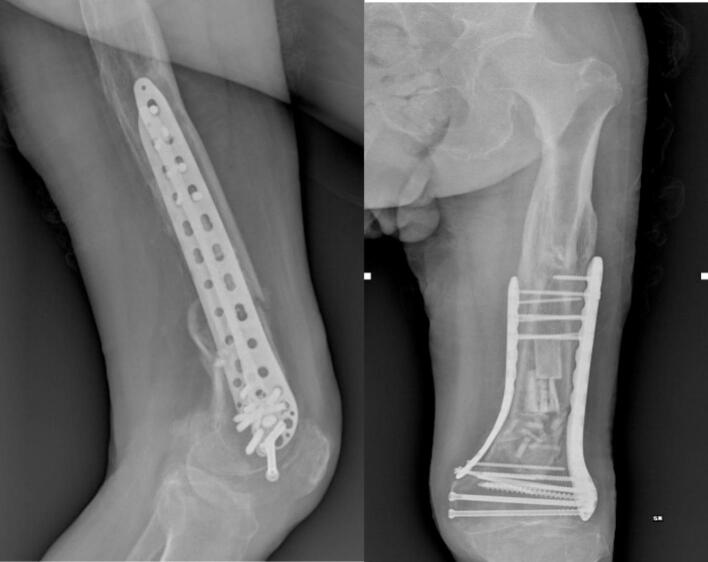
Control X-ray six months after surgery.
